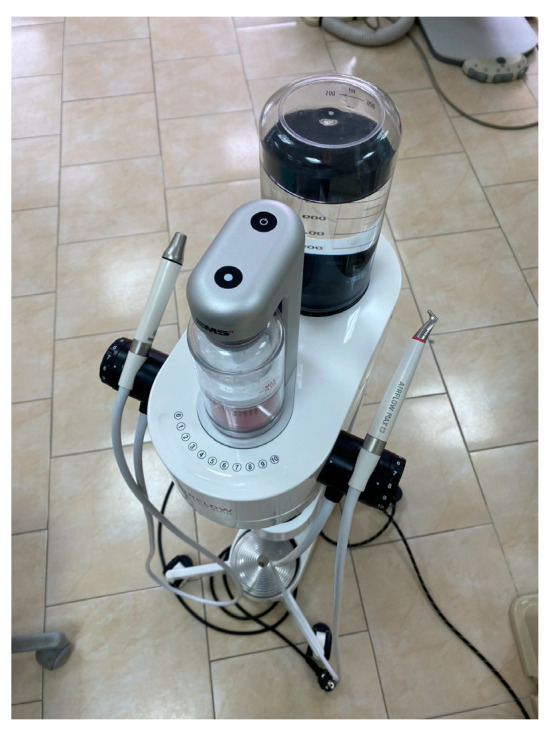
Combined ultrasonic and polishing device.
